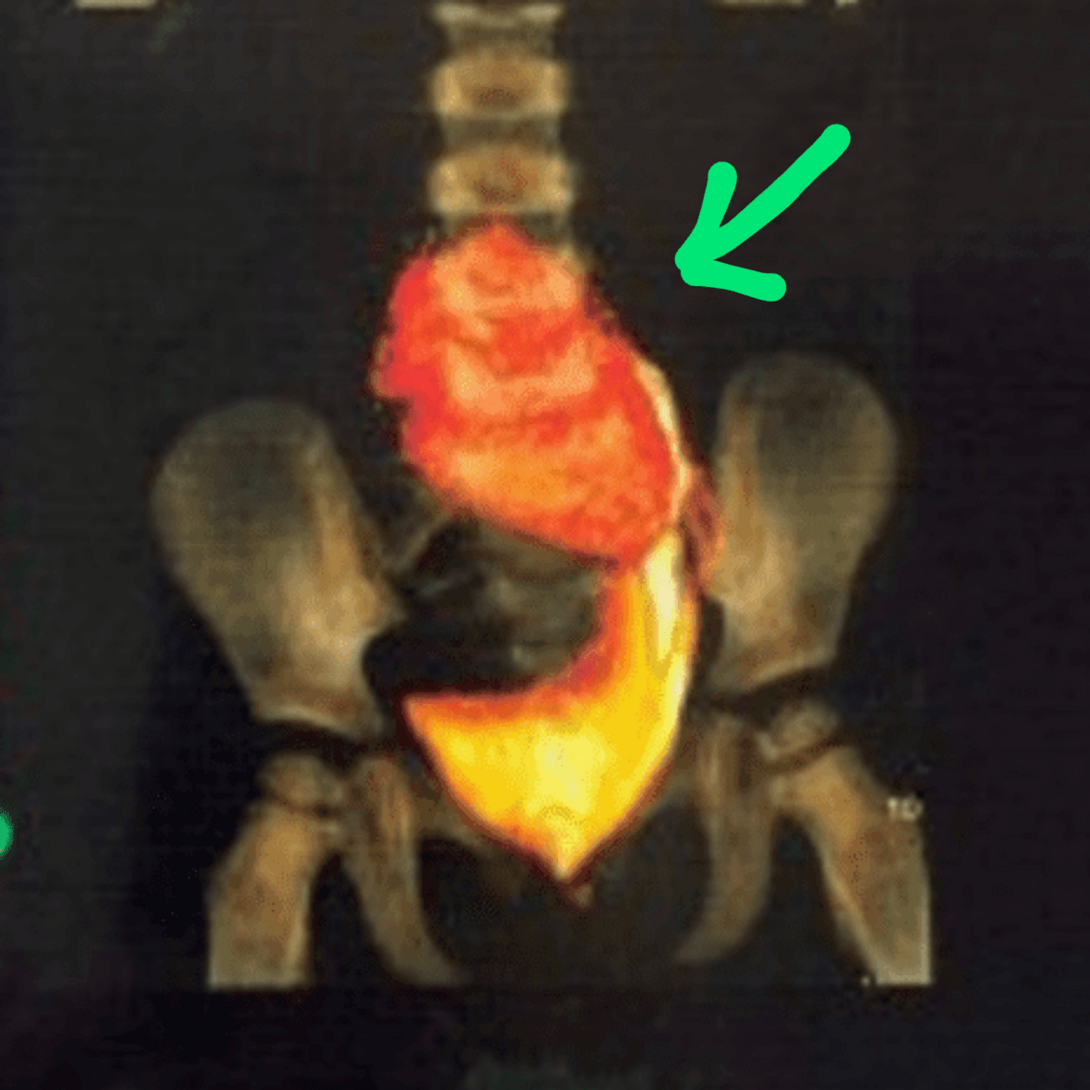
CT scan of the abdomen and pelvis showing the presence of a solitary right-sided ectopic kidney
